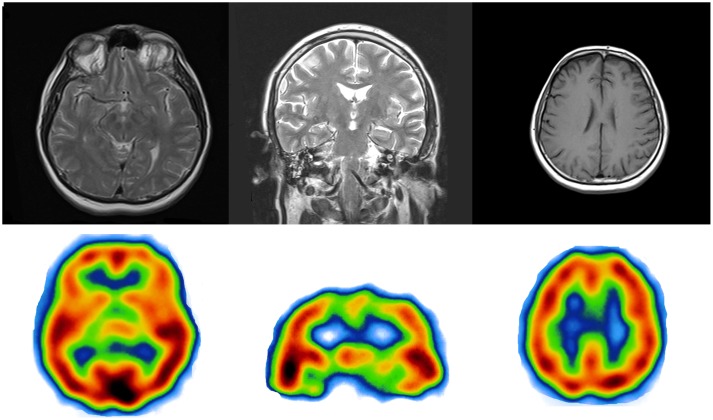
Results of MRI and SPECT examination of a control subject. Magnetic Resonance Imaging and Single Photon Emission Computed Tomography demonstrating the normal scans of a control, age-matched patient with no signs of any neurodegenerative disorder.
